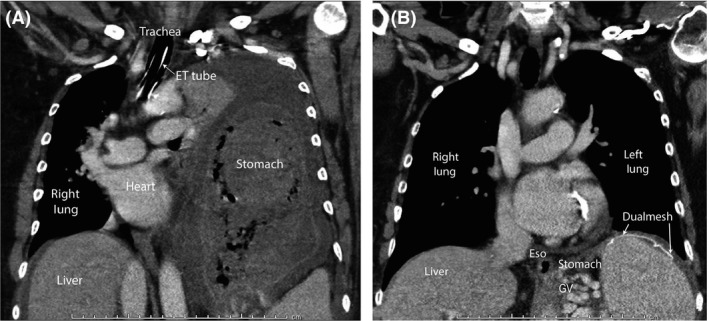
(A) Contrast CT chest image showing shift of mediastinal structures to the right, complete collapse of the left lung, near entirety of the stomach located within the left chest. (B) Contrast CT chest image postsurgical repair of diaphragmatic rupture with a Dualmesh and reduction of the hiatal hernia.
